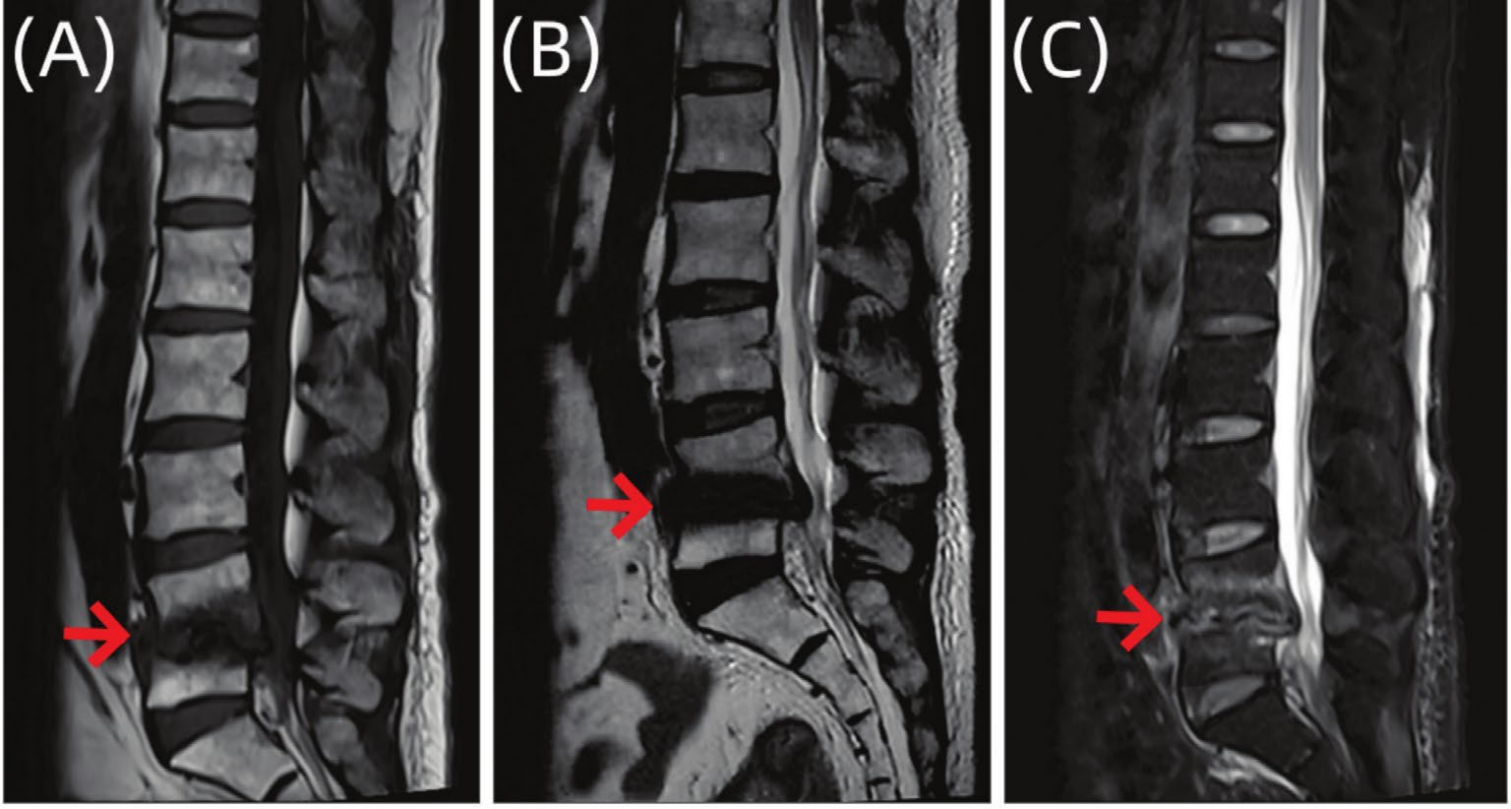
Sagittal MR images of the lumbar vertebrae of the patient. Imaging examination showed that the morphology of L4 and L5 vertebral bodies was irregular, and the edges of L4 and L5 vertebral bodies showed strips of abnormal signals, which were significantly reduced compared with (Figure 2) A–C.
